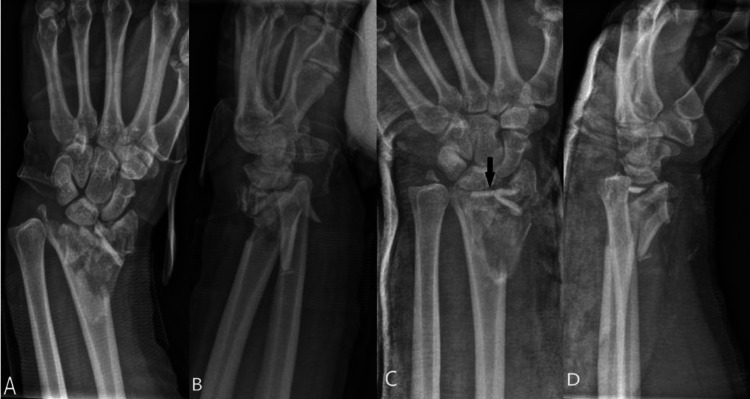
Antero-posterior and lateral radiographs on presentation A: Note the significant comminution and disruption of the radiocarpal joint surface. B: Note the dorsal subluxation of the radiocarpal joint and the significant comminution of the dorsal radial metaphysics. C: Anteroposterior radiograph after reduction. Note the significant comminution of the radial column and the large fragments of radial metaphysis present in the radiocarpal joint, as indicated by a black arrow. D: Lateral radiograph after reduction. The radiocarpal joint is still subluxed due to a lack of subchondral support, both palmarly and dorsally.
